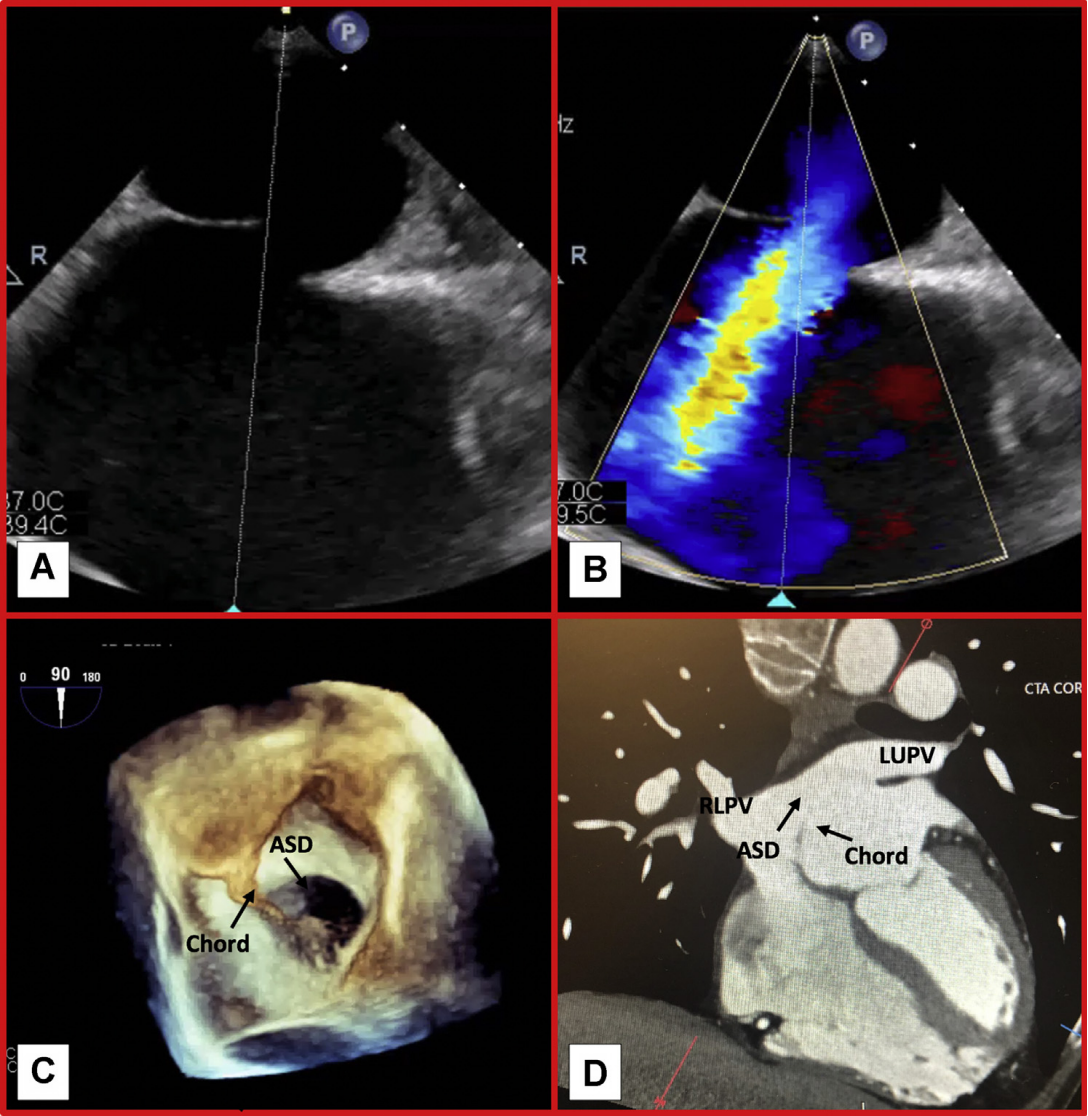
Tranesophageal Echocardiogram and Cardiac Computerized Tomography Findings Two-dimensional transesophageal echocardiography views with (B) and without (A) color Doppler revealing the malaligned atrial septal defect (ASD). (C) 3-Dimensional image of left atrial perspective of ASD along with the membranous chord. (D) Cardiac computerized tomography confirming attachment of membranous chord to the left atrial wall. LUPV = left upper pulmonary vein; RLPV = right lower pulmonary vein.
